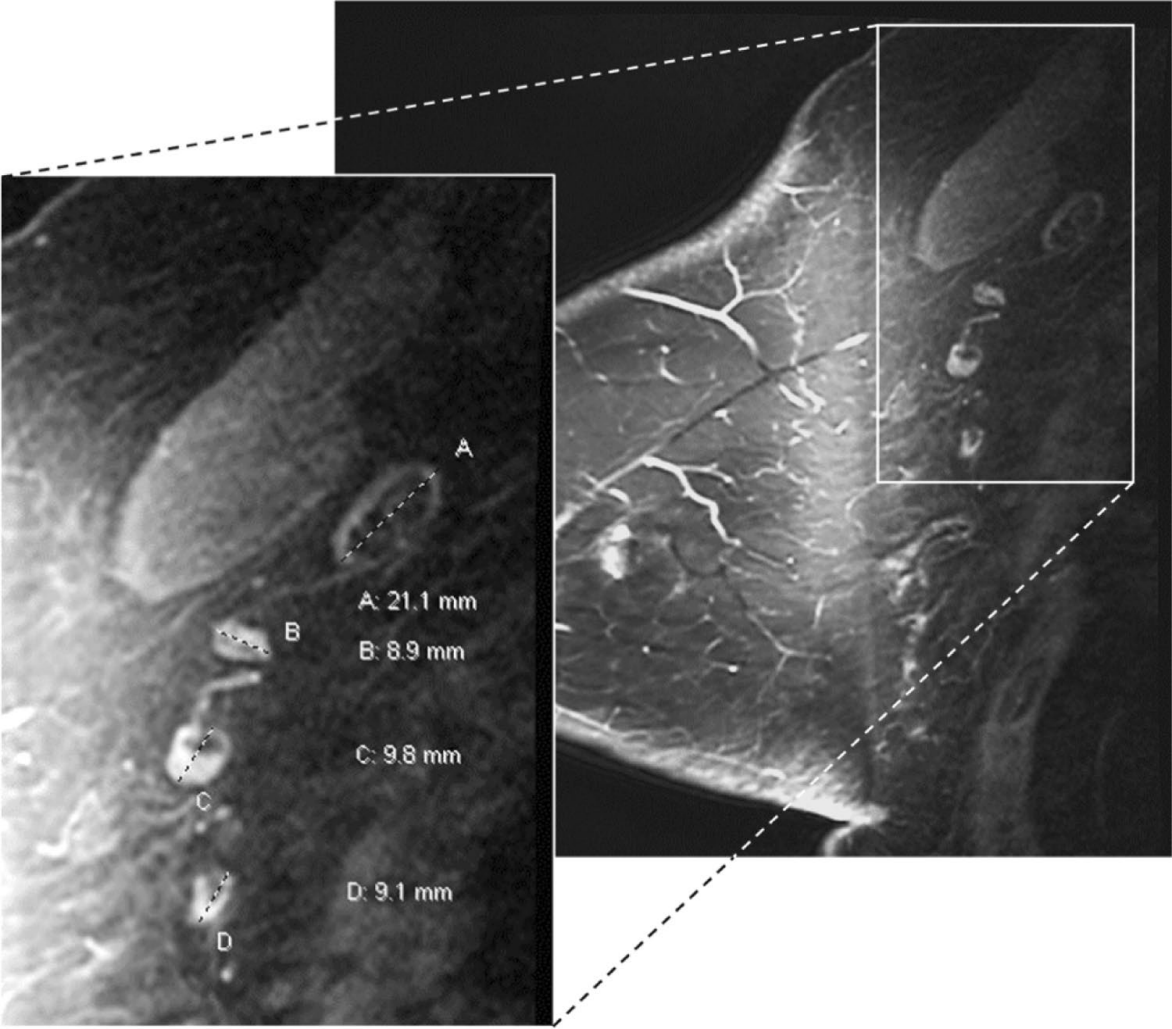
Variable axillary lymph node size and morphology on breast MRI. 65 y/o female with ER+ HER2− infiltrating ductal cancer of the left breast showing benign variable lymph node size and morphology in the right axilla contralateral to the known breast cancer. Contrast-enhanced fat-saturated sagittal MRI image of the right breast, and enlarged view of the right axilla demonstrate variable nodal size and morphology. Fat-enlarged node with expanded fatty hilum measures 21 mm in length (A) while normal nodes with small fatty hila measure less than 10 mm (B, C, D). The largest visible axillary node of 21 mm was chosen as the index node for the analysis in our study
